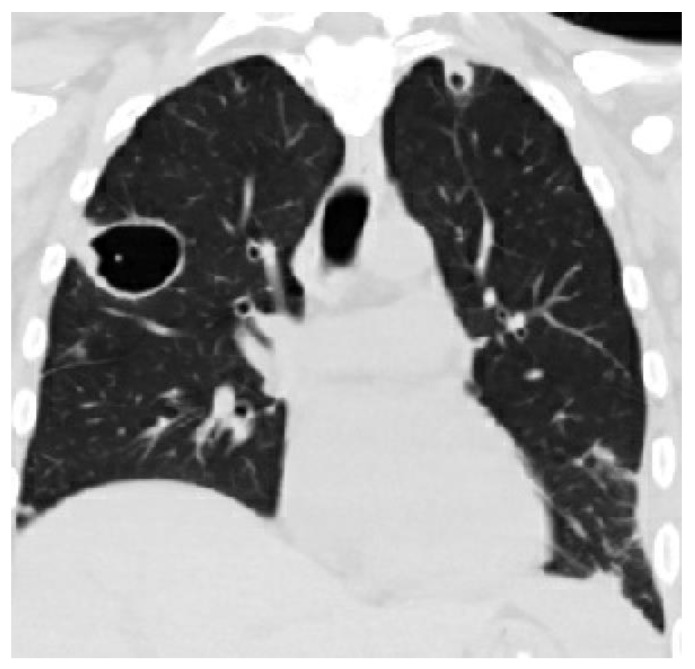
A CT scan of a patient with radiographic evidence of septic emboli.
